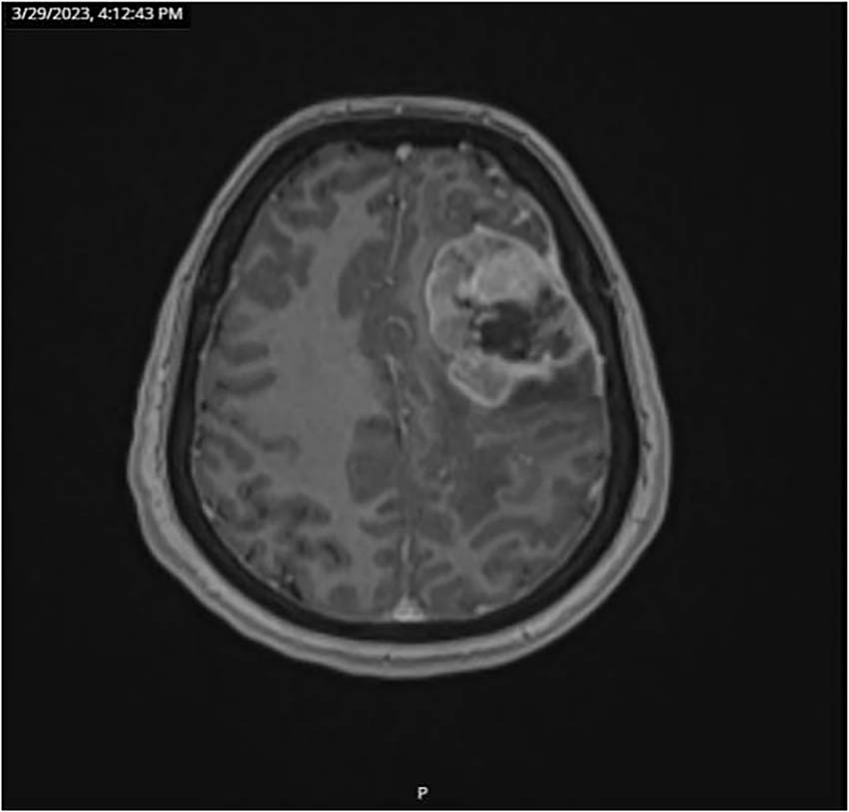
Brain MRI with gadolinium injection on 29 March 2023.
